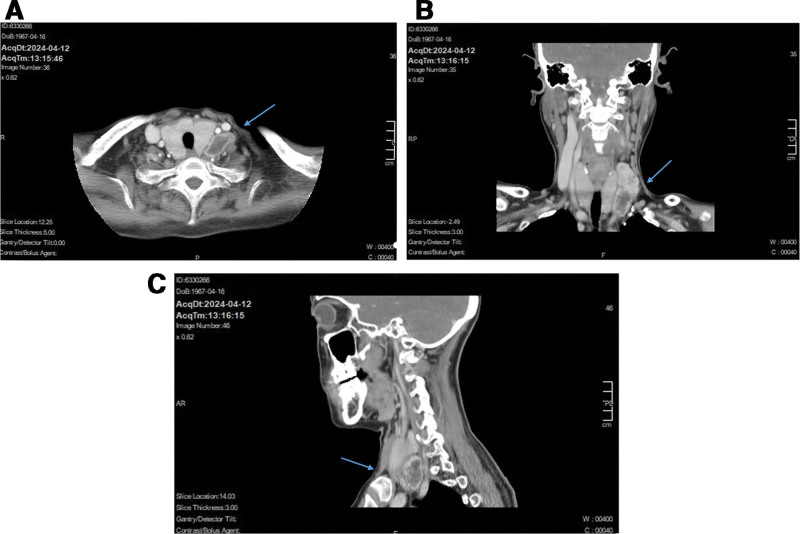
CT examinations. (A) Enhanced CT scan of the neck in horizontal position. (B) Enhanced CT scan of the neck in coronary position. (C) Enhanced CT scan of the neck in sagittal position. The arrows indicate the large cystic-solid mass (parathyroid carcinoma) located between the left carotid sheath and the thyroid gland.
